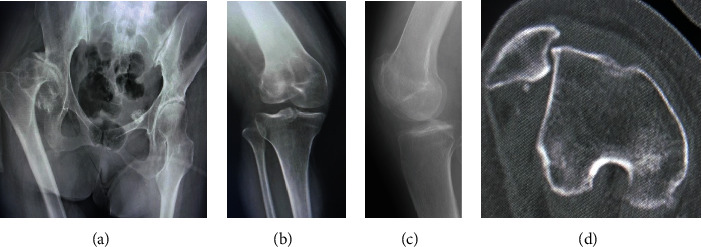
(a) Preoperative X-ray examination of the hip. (b) Preoperative X-ray examination of the right knee; anteroposterior view. (c) Preoperative X-ray examination of the right knee; lateral view. (d) Preoperative computed tomography of the right knee.
